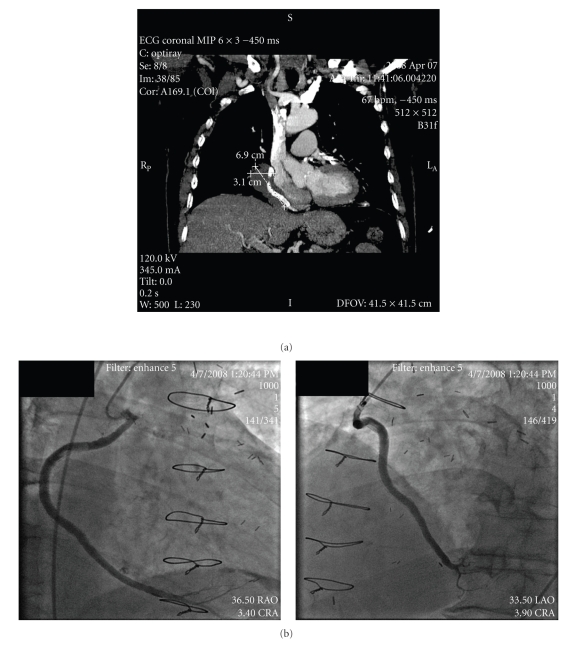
(a) CT of the chest in April 2008 revealing aneurysm size (3.1 cm × 6.9 cm) has remained unchanged. (b) Coronary angiography in April 2008 in both RAO and LAO views revealing patent stents and no evidence of leak through the covered stents into aneurysm.
